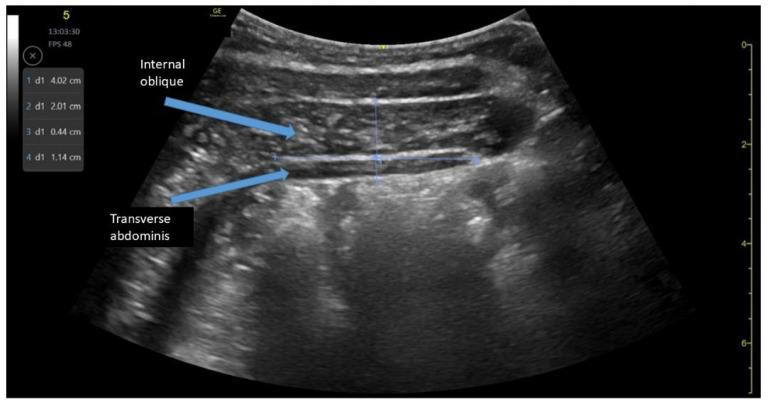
Ultrasound assessment of abdominal muscles at rest and muscle thickness measurements.
